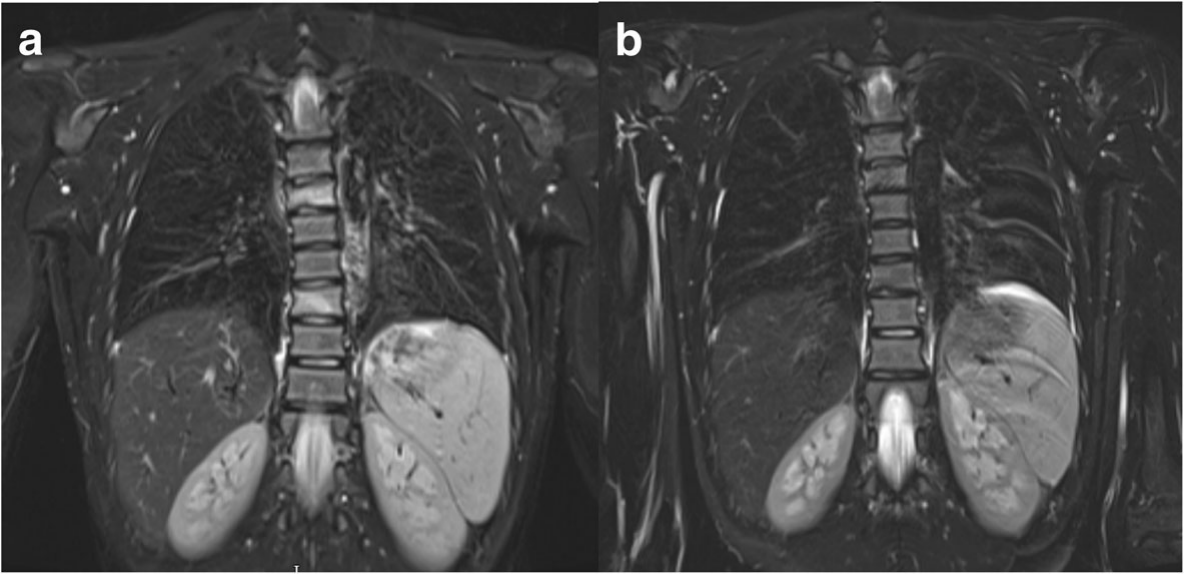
Pre and post-pamidronate WB-MRI. a The coronal STIR image shows high signal predominantly of the T6 and T9 vertebral bodies consistent with bone oedema. b Almost complete resolution of high signal changes in keeping with treatment response after four cycles of pamidronate. There is moderate loss of height at T6 and slight loss of height at T9 vertebral bodies
